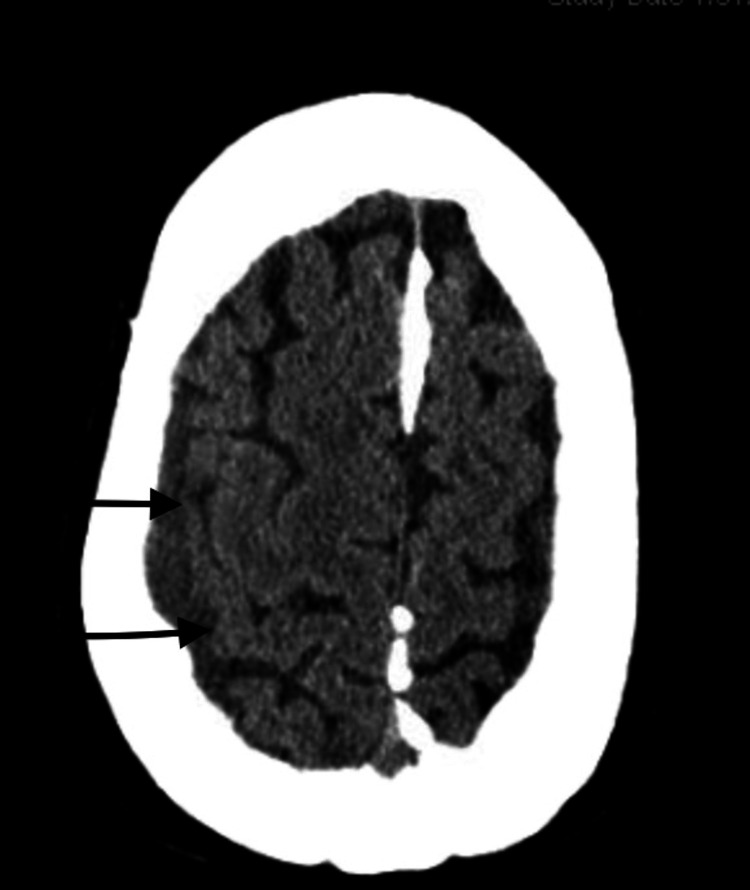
Computed tomography scan of the brain showing a hypodense collection (see black arrows) in keeping with a chronic, right-sided subdural hematoma
